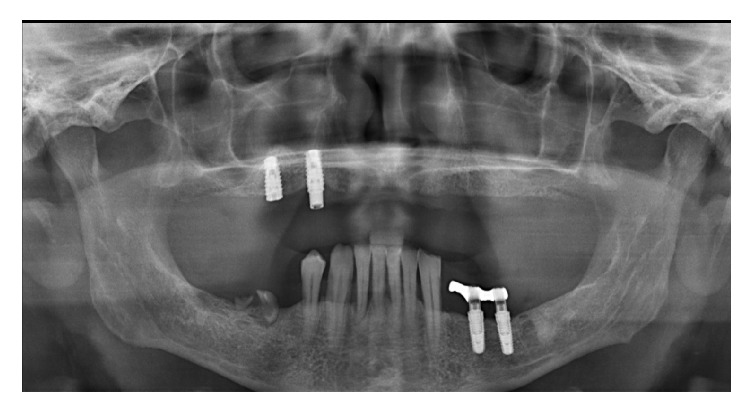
A preoperative panoramic radiograph.
